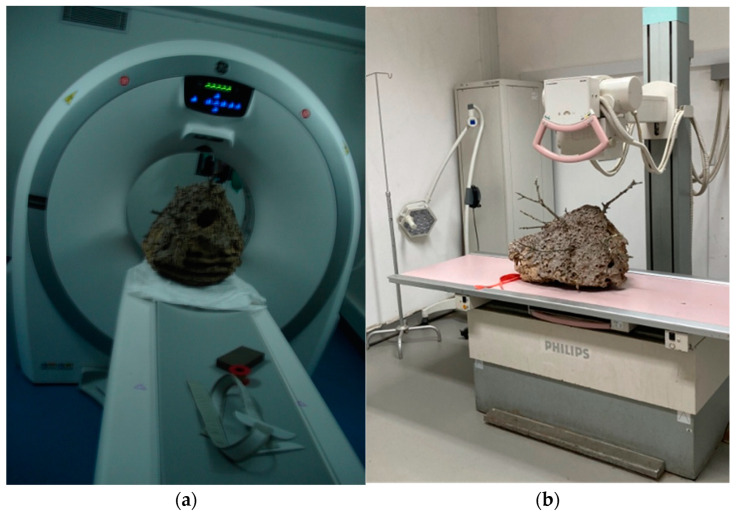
Equipment used for the examination of the Asian Hornet nest: (a) computed tomography (CT) assessment; (b) computed digital X-ray device.
